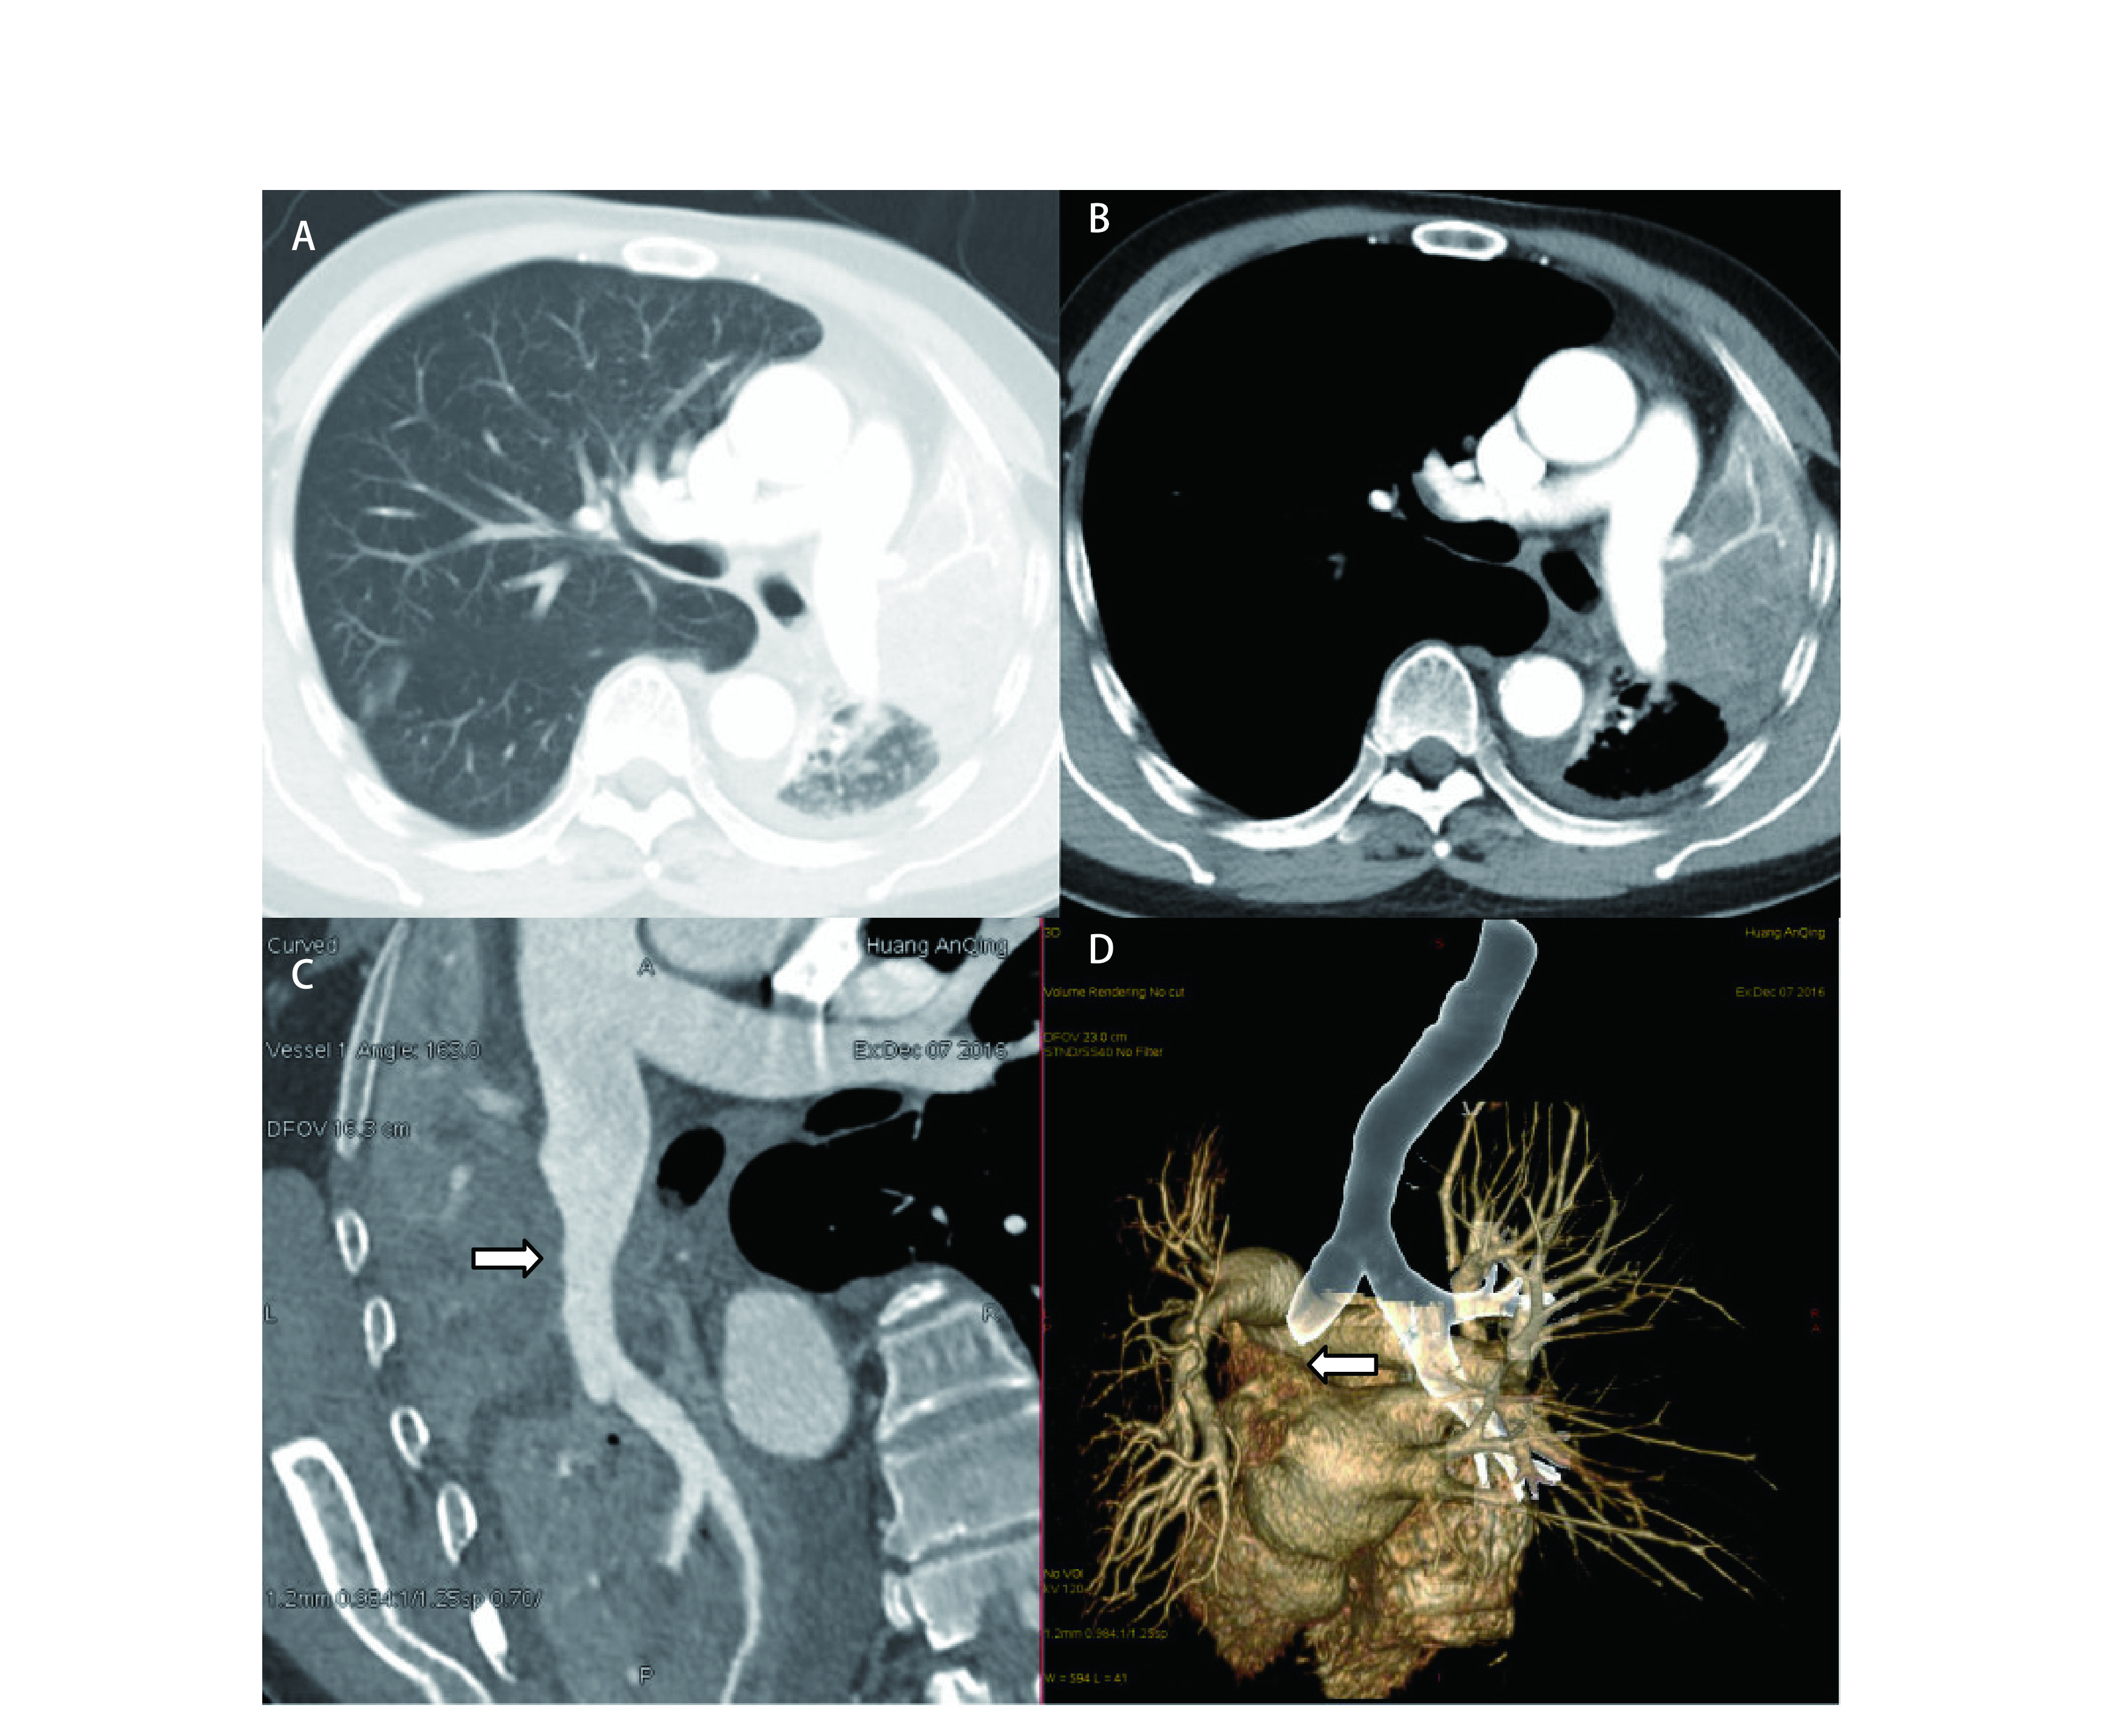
胸部增强CT检查。A：肺窗, 左主支气管堵塞, 左肺上叶肺不张, 肺实变, 左侧胸腔缩小; B：纵隔窗, 肿瘤包绕左肺动脉干, 肿瘤61 mm×53 mm×65 mm; C：矢状面重建显示肿瘤侵犯肺动脉达斜裂水平(白色箭头所示); D：三维重建显示左主支气管折断(白色箭头所示)。 Thoracic enhanced CT scanning. A: Pulmonary window: The left upper lobe atelectasis and consolidation, the left thoracic cavity reduction, because the left main bronchus blocked by tumor; B: Mediastinal window: The tumor surrounds the left pulmonary trunk. The tumor size is 53 mm×61 mm×65 mm; C: The sagittal reconstruction of the lung showed that the tumor invades the left pulmonary artery (as indicated by the white arrow); D: CT 3D reconstruction shows the left main bronchus broken (as indicated by the white arrow). CT: computed tomography.
